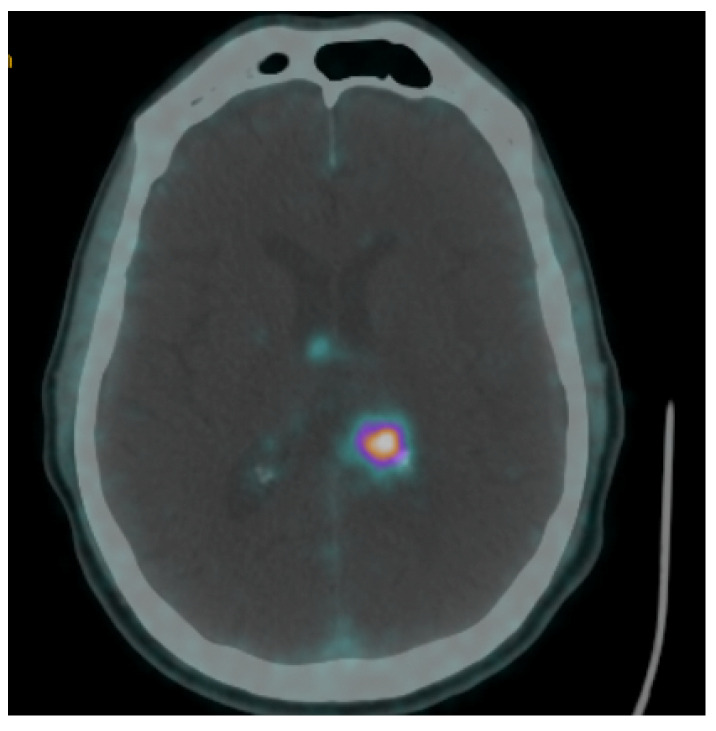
[^68^Ga]Ga-DOTATOC PET/CT scan showing a left intraventricular lesion with high tracer uptake in a patient with a history of carcinoid. GK treatment was planned by contouring the margins of the lesion with tracer uptake.
